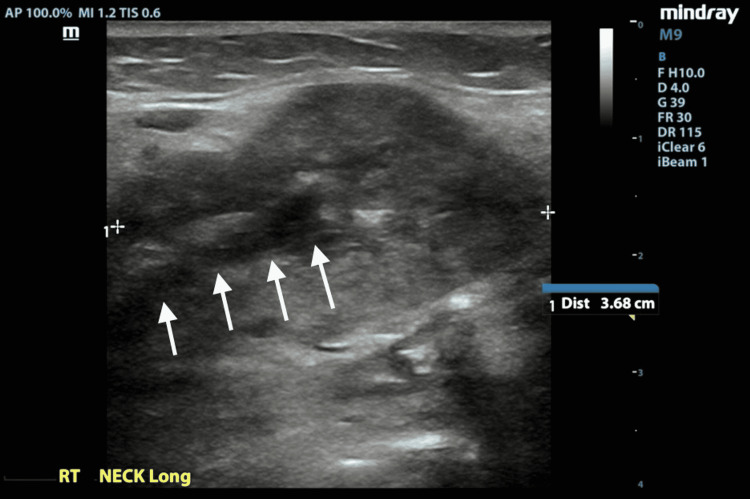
POCUS of the right submandibular gland: longitudinal view of the enlarged right submandibular gland with heterogeneous echotexture and dilated Wharton’s duct (see arrows). POCUS: point-of-care ultrasound (POCUS)
